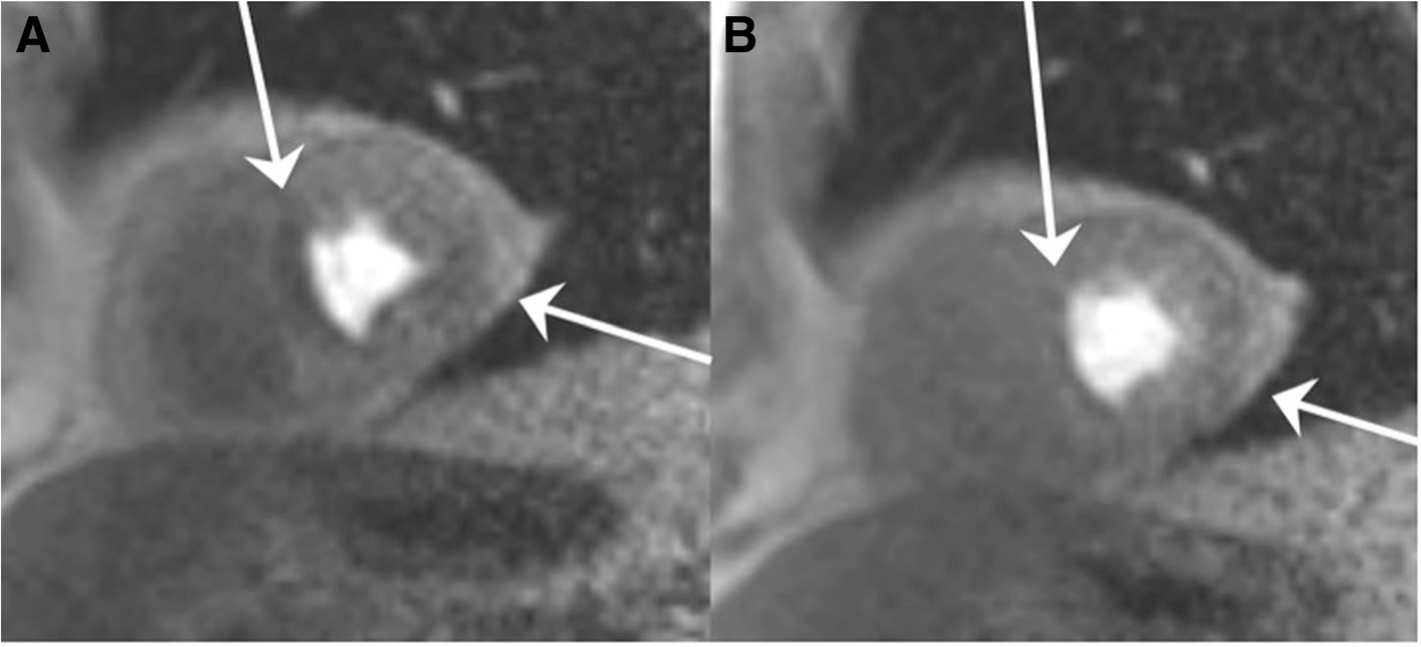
Perfusion defect on coronary magnetic resonance imaging. Coronary magnetic resonance imaging during pharmacological stress induced hyperemia (a) and at rest (b). The patient has an anteroseptal and lateral reversible perfusion defect in the midcardial segments of the left ventricle
